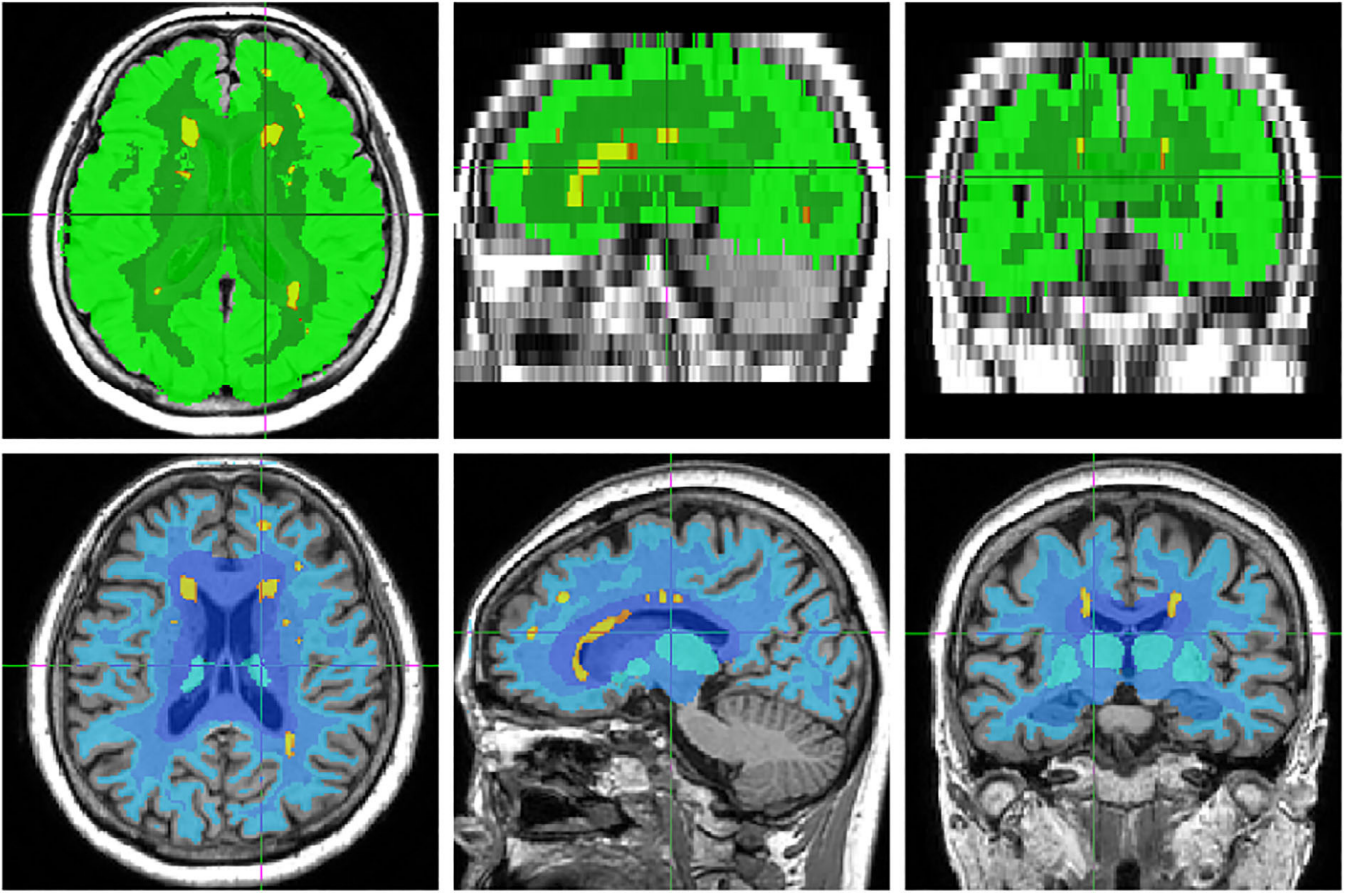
Two separate ConvNets were tasked with generating relevant regions of interest (ROIs) represented as color shades for 2D FLAIR (top, greens) and for T1 images (bottom, blues). The FLAIR model, used for temporary usage, was only approximate because of the poor resolution and lack of clearly visible structures. The final model, on T1, was intended to classify lesions (identified and depicted as yellow patches). Still, the hard borders of the ROIs make the current aggregation method perfectible.
